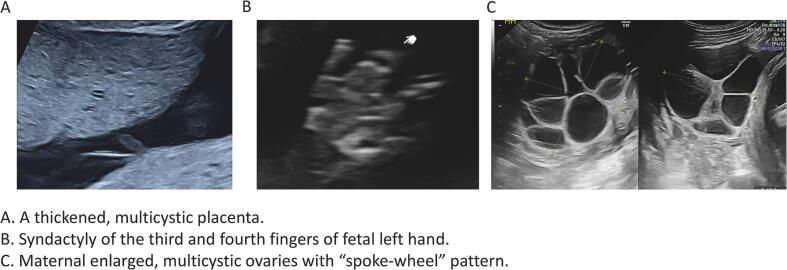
Ultrasound screening at 19 weeks of gestation.
